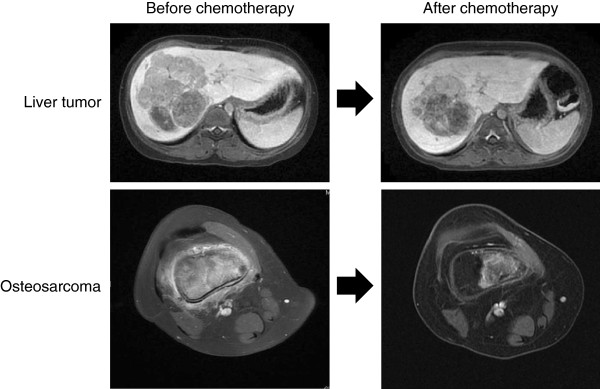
Reduced tumor masses in the liver and bone after chemotherapy. Magnetic resonance imaging (MRI) revealed that the sizes of both the liver tumor and the osteosarcoma were significantly reduced by the combination chemotherapy
